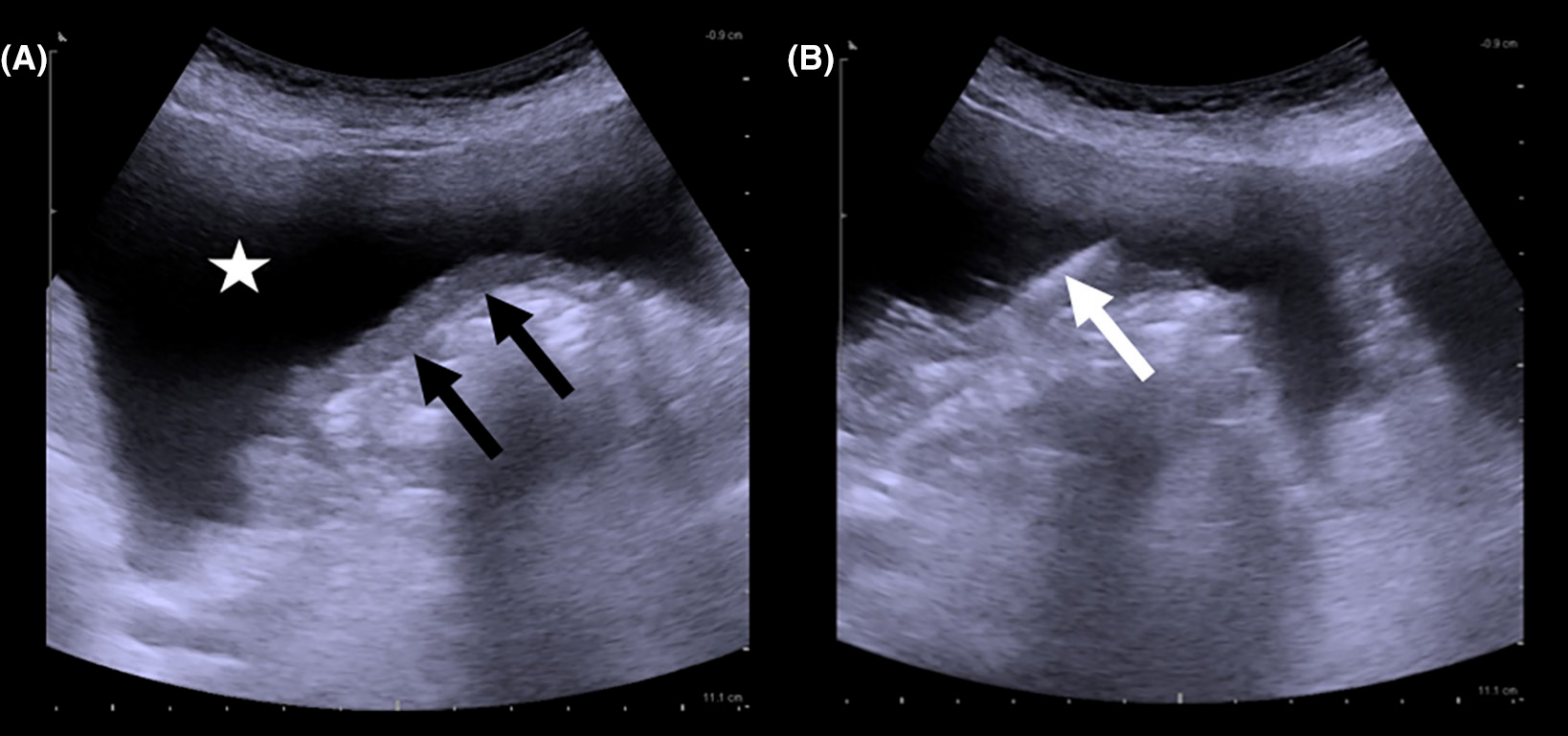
A 76‐year‐old woman presented with no prior history of cancer who was hospitalized with abdominal distension and an omental mass. Ultrasound images (A, B) show a thickened omentum (black arrows) and an 18‐gage core biopsy needle (white arrow) with ascites (asterisk). Her pathological diagnosis was high‐grade ovarian serous adenocarcinoma metastasis.
